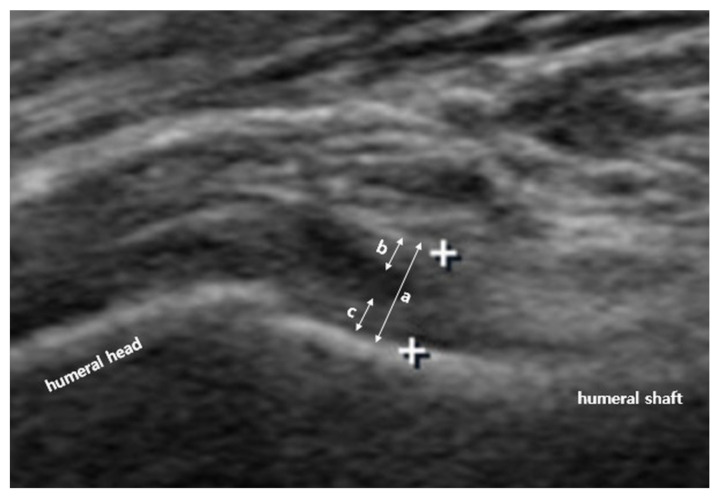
Ultrasonographic measurement of inferior joint capsule (IJC) thickness. IJC thickness was defined as the distance from the cortex of the humerus to the outer margin of the capsule at the widest portion of the capsule. IJC thickness (a) includes both the glenoid side (b) and humeral side (c).
